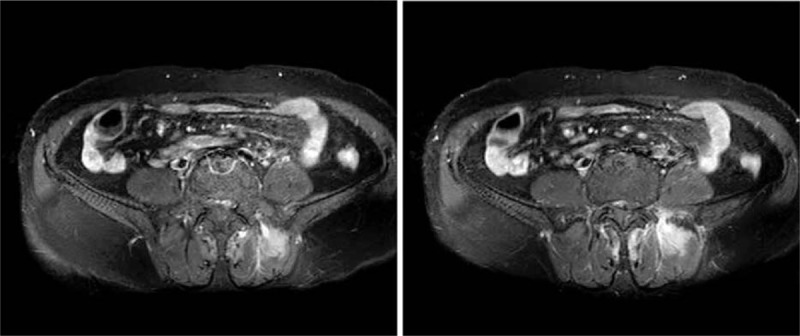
Magnetic resonance imaging (MRI) of the sacroiliac joints showed diffuse hyperintense signal on gadolinium-enhanced, T1-weighted, fat-saturated images in the left erector spinae muscles which indicate reactive myofascitis.
